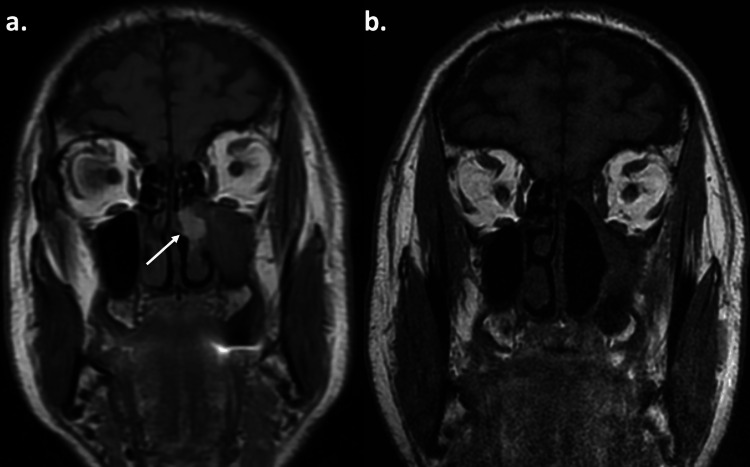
Before and after excision of the left paranasal sinus metastasis (a) Magnetic resonance imaging (MRI) of the head (T1-sequence), revealing a tumor (pointed by the white arrow) in the left infundibulum. (b) Postoperative MRI of the head (T1-sequence) showing the left median maxillectomy, ethmoidectomy and resection of the middle turbinate.
